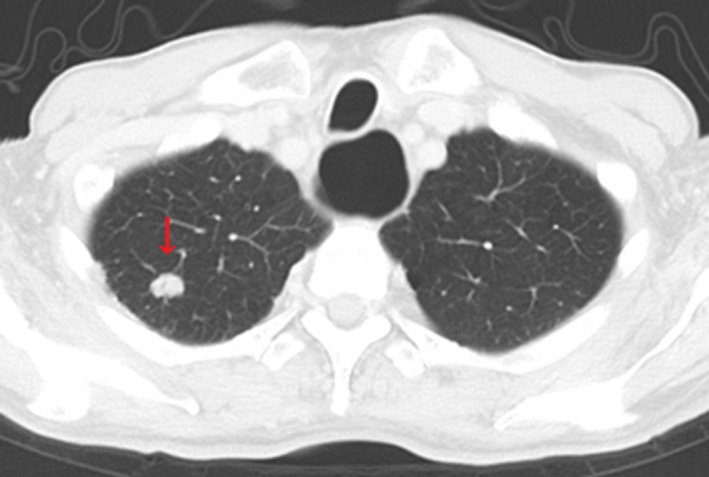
Chest computed tomography scan findings. A lung tumor was detected in the upper lobe of right lung 6 years after the surgery (arrow)
